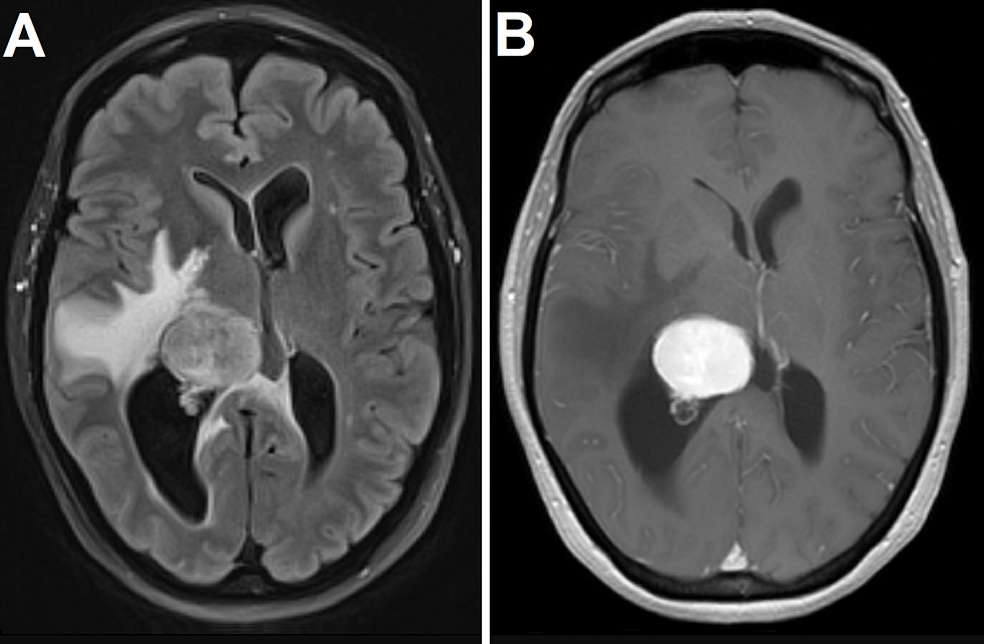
Magnetic resonance imaging of the brain with fluid-attenuated inversion recovery (A) and gadolinium-enhanced T1-weighted (B) images demonstrating an avidly contrast-enhancing, well-demarcated intraventricular tumor with associated vasogenic edema.
